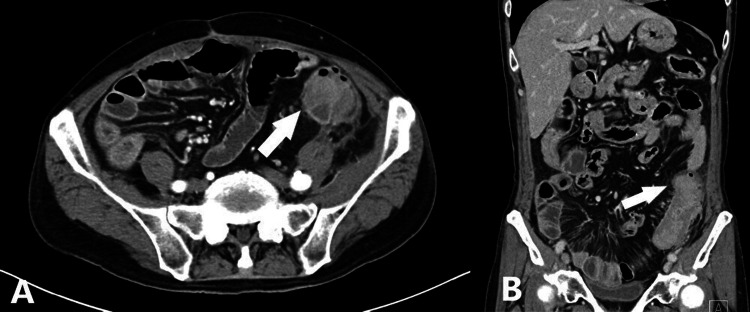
Abdominal contrast-enhanced CT scans, axial view (A) and coronal view (B) Diverticula, concentric wall thickening, and pericolic fat stranding were observed in the descending colon (white arrow).
